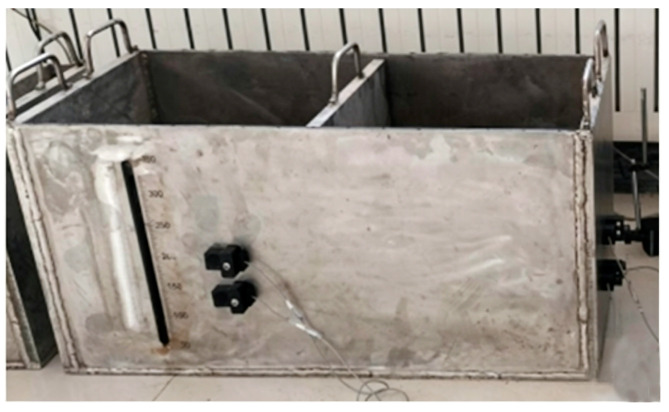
Installation positions of the ultrasonic transducers.
